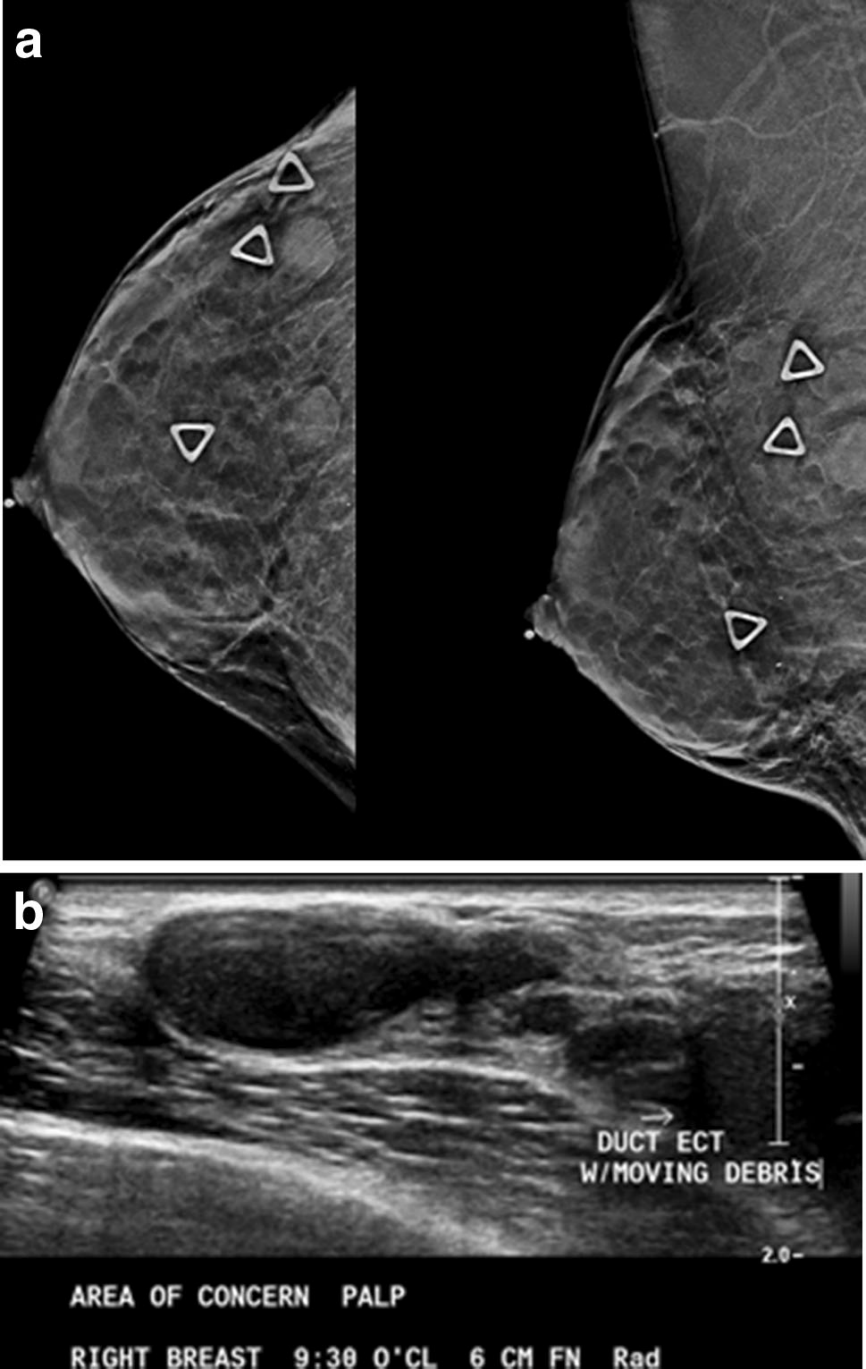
A 58-year-old transgender woman on hormone treatment complains of lumps in her breast. a CC and MLO mammography views show triangle-shaped skin markers over palpable masses. b Ultrasound shows benign duct ectasia which correlates with the palpable masses
